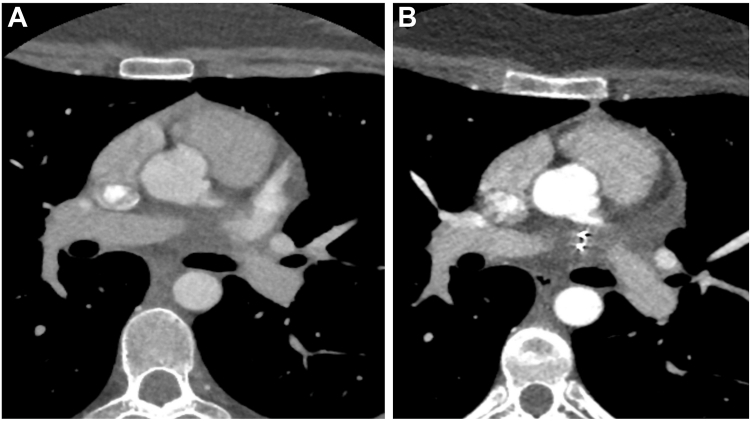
Axial computed tomography cardiac angiogram before and after left atrial appendage (LAA) closure. A: Preclosure: the LAA is well opacified with contrast, indicating normal blood flow. B: Postclosure: a radiopaque clip is visible at the LAA ostium with no contrast filling, consistent with successful LAA exclusion.
